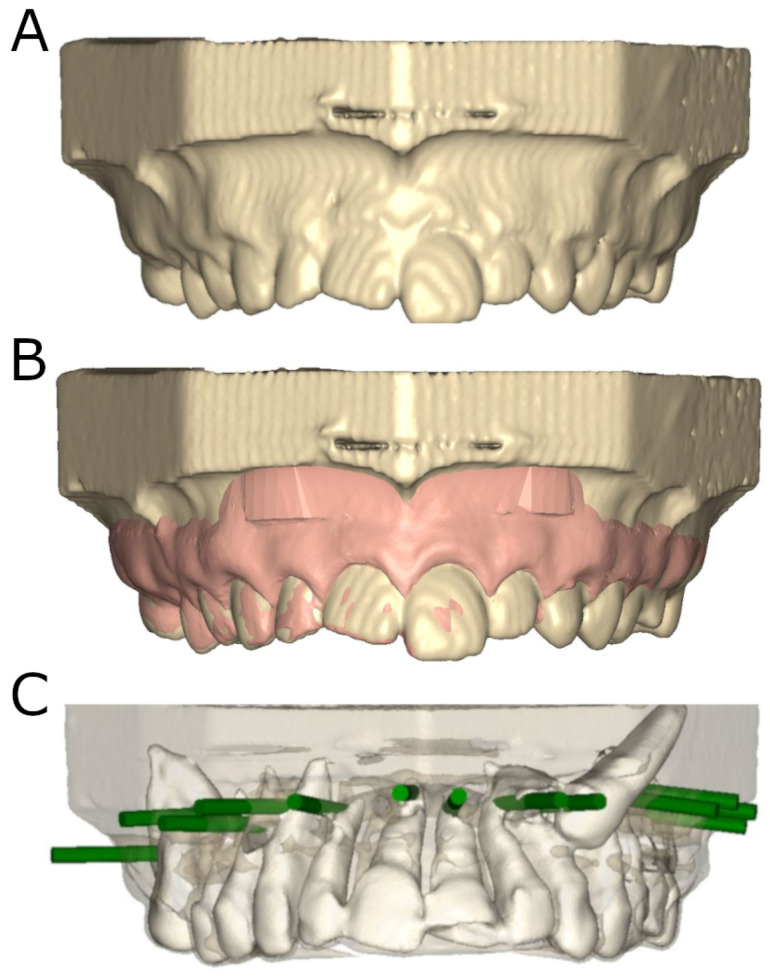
(A) Image from CBCT scan, (B) alignment of CBCT scans with STL digital files, (C) front view of virtually planned preparations of osteotomy sites (green cylinders), with surrounding tissues.
